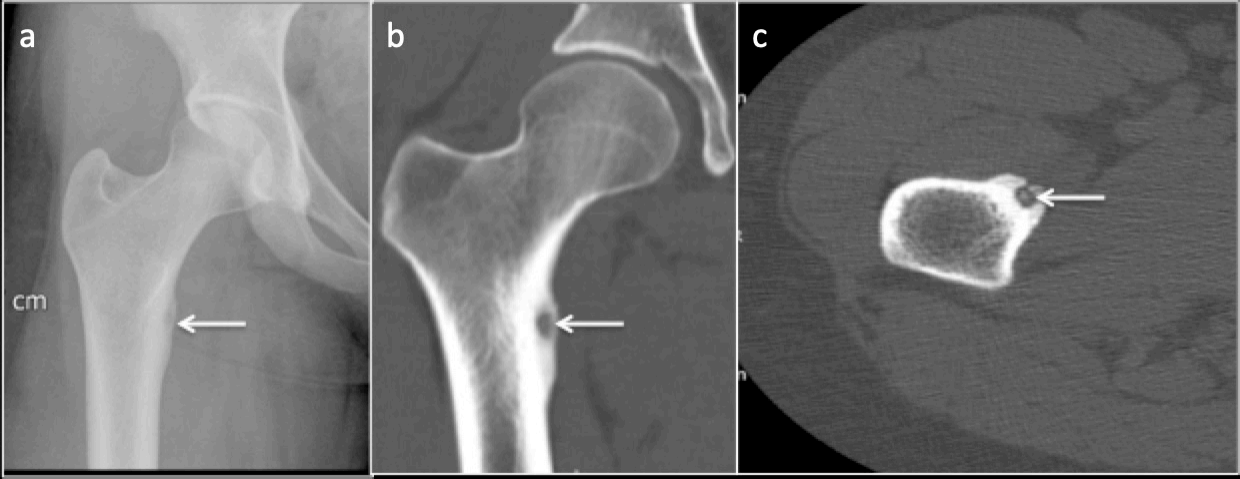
Patient presenting with hip pain. (a) Radiograph demonstrating central lucency (arrow) with surrounding sclerosis and cortical thickening along the medial cortex of proximal femur resembling a fatigue fracture. (b) Coronal and (c) Axial CT images demonstrating central nidus and surrounding sclerosis and cortical thickening indicating that the lesion is osteoid osteoma.
